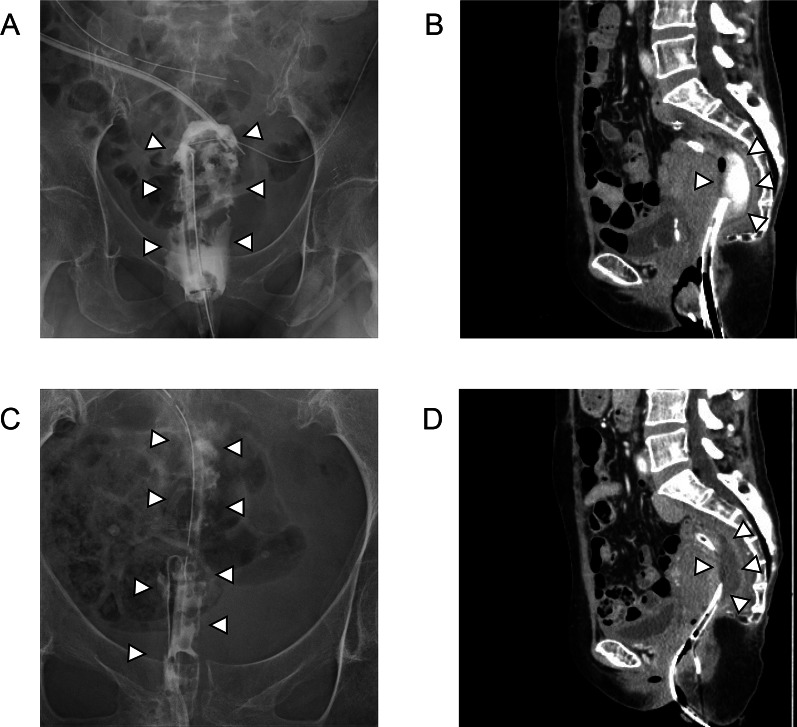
A, B Radiographic contrast examination (A) and CT imaging (B) findings after the first surgery. A Transanal drainage tube was added to the pelvic abscess cavity. The abscess cavity was contrasted by gastrografin. B CT image findings indicated a pelvic abscess cavity. C, D Radiographic contrast examination (C) and CT imaging (D) findings before reoperation. C, D Pelvic abscess cavity decreased but persistently remained by radiographic contrast examination (C) and CT (D). White arrowheads indicate the abscess cavity
